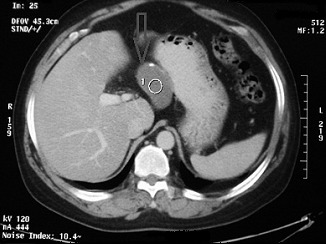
Abdominal computed tomography scan of the patient, revealing the duplication cyst in the proximity of the gastric lesser curvature.
